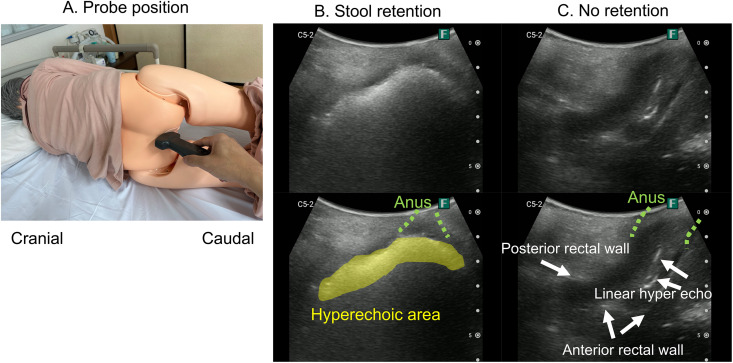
Probe position and rectal ultrasound images in the transgluteal cleft approach scanning method. (A) Probe position. A mannequin (or patient) is placed in a left lateral recumbent position with the knees flexed to create a space between the coccyx and the anus. The probe was applied to this area with a longitudinal scan. (B) Ultrasound image when there is fecal retention. Feces in the lower rectum are observed as hyperechoic areas. (C) Ultrasound image when there is no fecal retention. A hyperechoic line is observed when feces are not accumulated, and even the anterior rectal wall is observed. All photographs and ultrasound frames are the authors’ own; no third-party copyrighted material or logos were reused.
